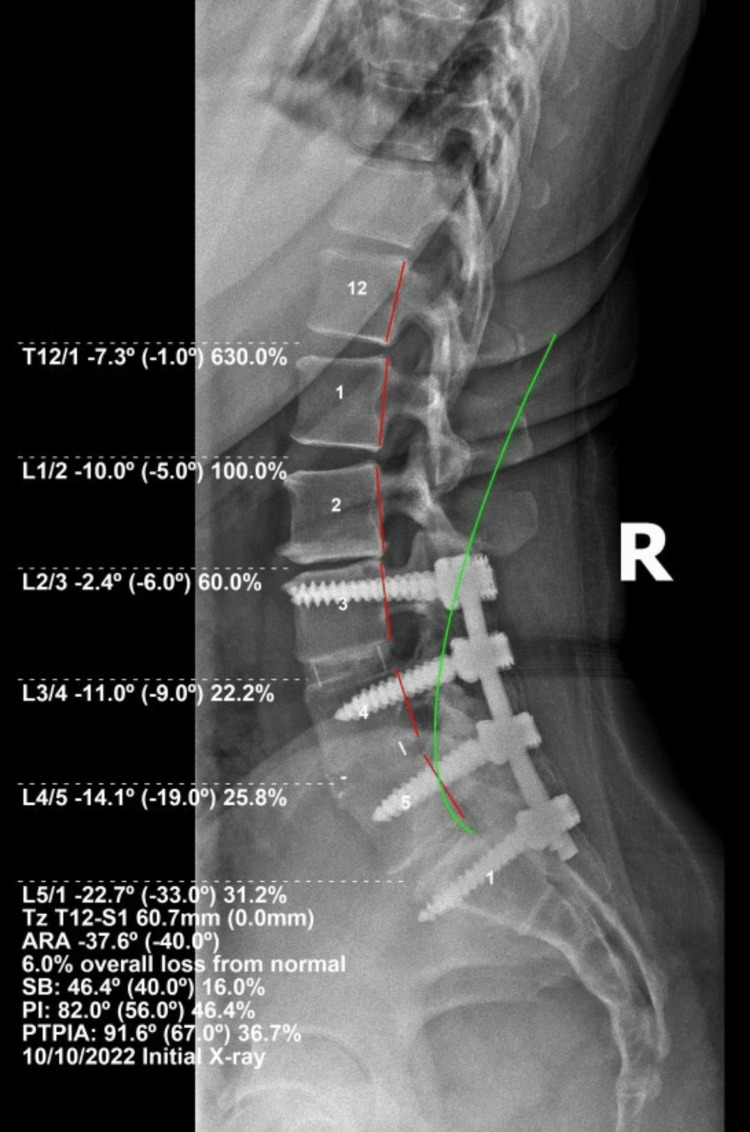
Initial lateral lumbar radiograph with PostureRay Biomechanical assessment.
